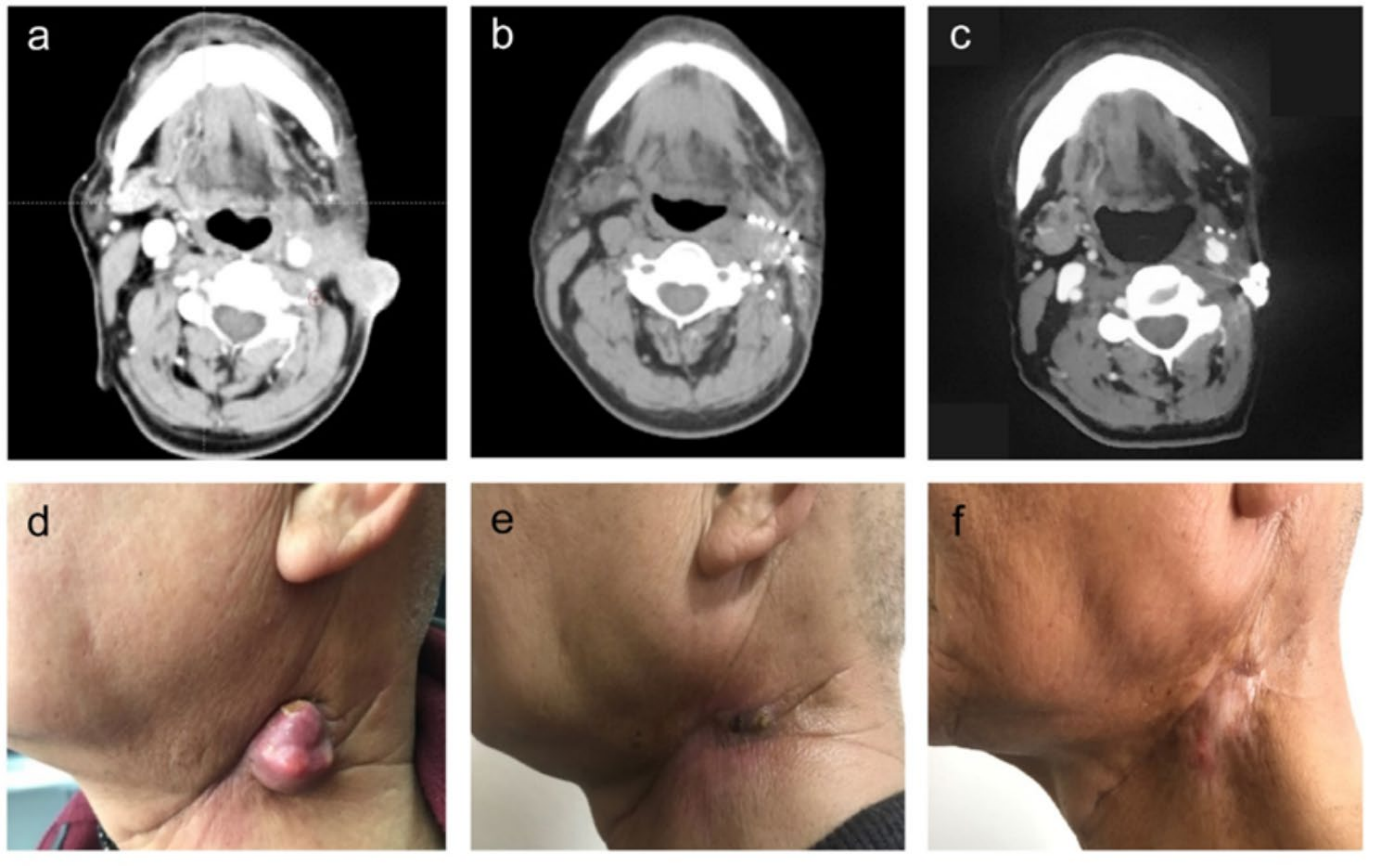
The therapeutic efficacy of CT-guided radioactive iodine-125 seed implantation Figure (a-c) showed CT images of the tumor in preoperation, 3-month postoperation, and 18-month postoperation in a case of squamous cell carcinoma of the tongue with cervical lymph node metastasis. Figure (d-f) showed the tumors of preoperation, 3-month postoperation, and 18-month postoperation by gross photos, respectively
